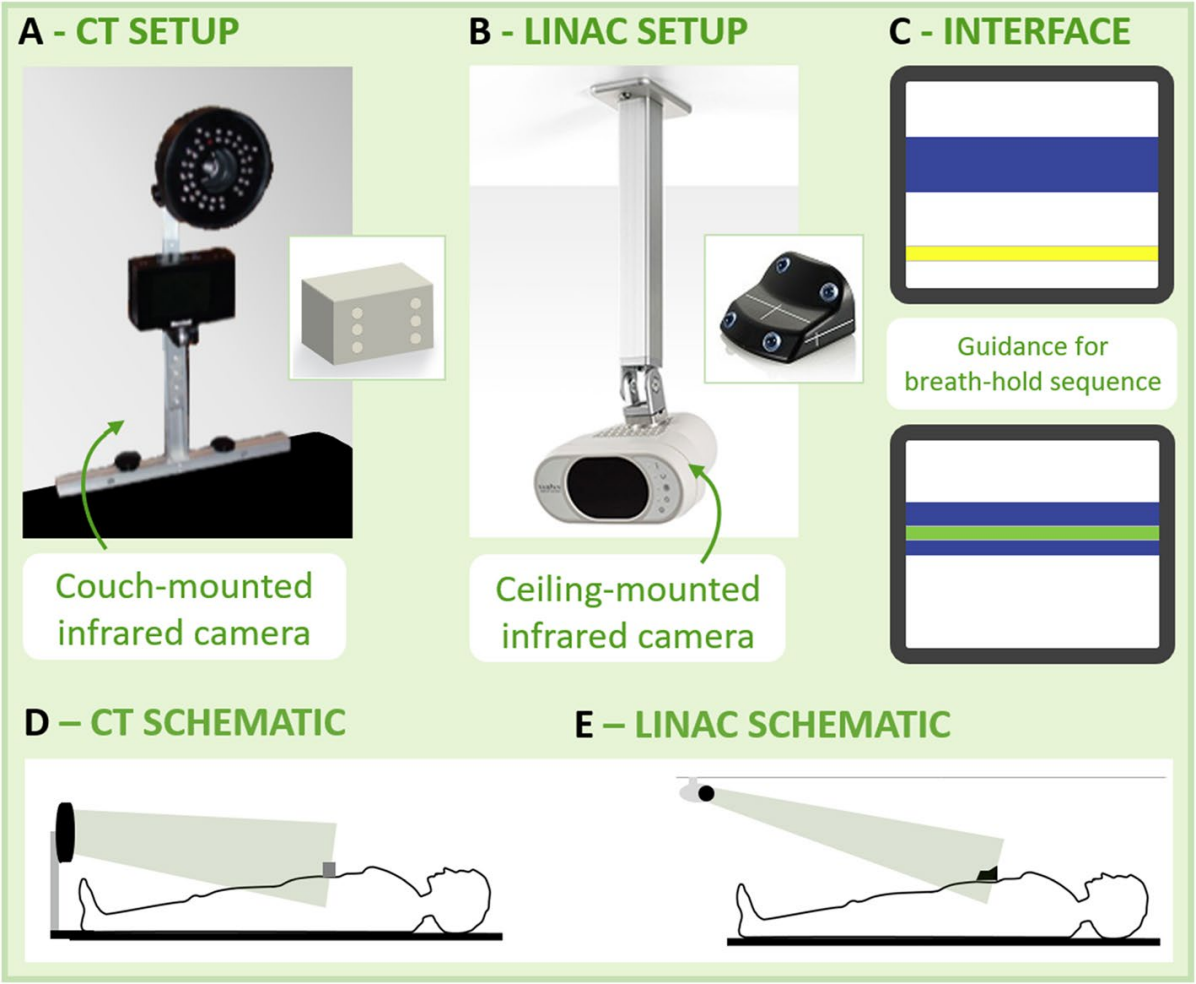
The RPM and RGSC systems. A RPM system used at CT. B RGSC used during linac treatment. C Patient screen with breath hold position visualisation. D Camera and marker block setup at CT. E Camera and marker block setup at treatment
